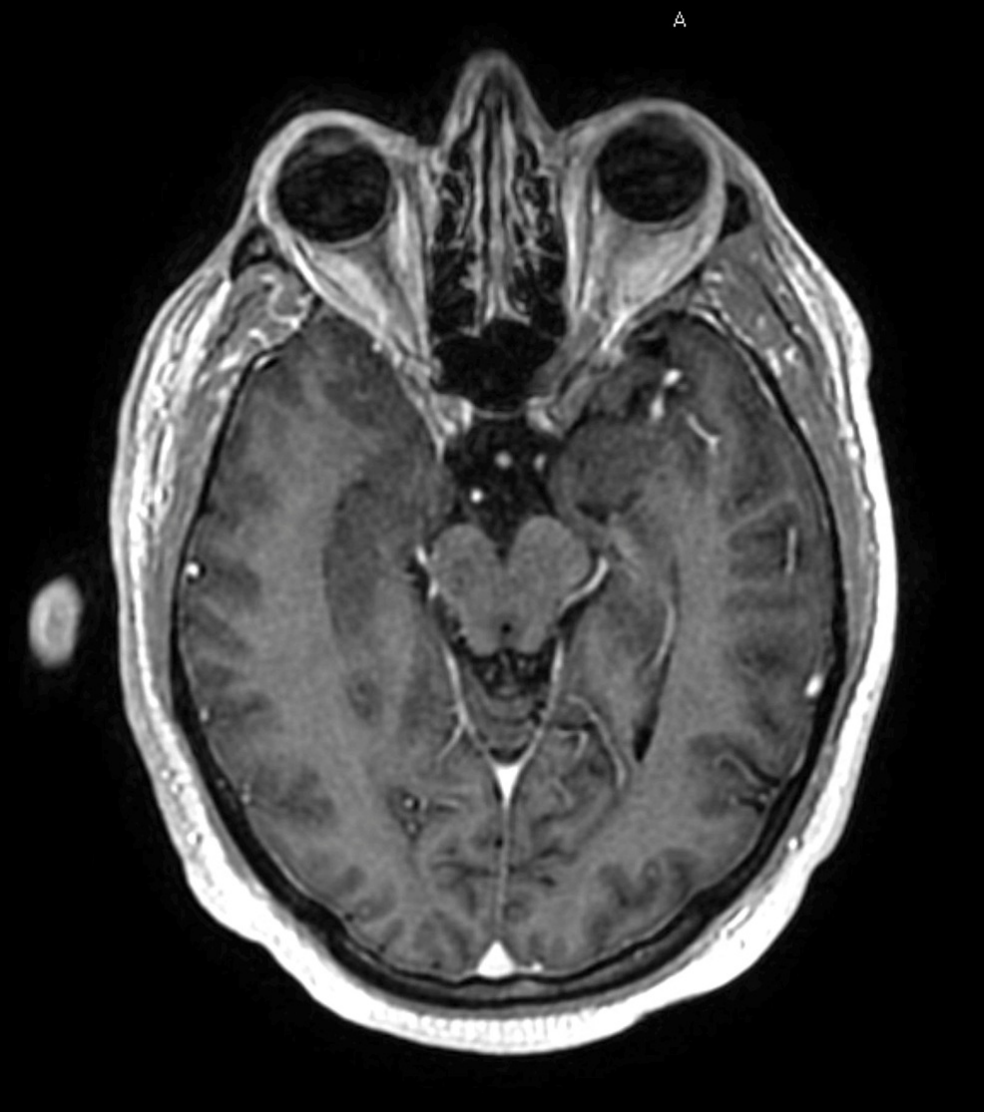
Axial head MRI T1 post-contrast at the level of the optic nerves showing no abnormal enhancement or signs of leptomeningeal disease
